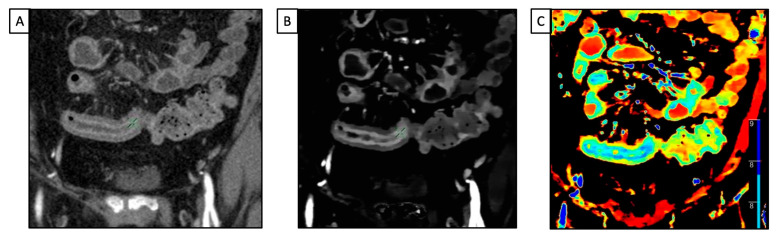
A 49 y-o male with known Crohn’s disease, diffuse abdominal pain, and suspected relapse of disease underwent abdominal CT. (A) Conventional CT images on the coronal plane acquired after intravenous contrast media injection show a poor layered enhancement appearance of the distal ileum; (B) iodine map enhances iodine’s uptake by the mucosa layer, and the hypoattenuating appearance of the submucosa one, consistent with edema; (C) the Z-effective map allows us to define the pattern of enhancement due to the atomic number of iodine.
